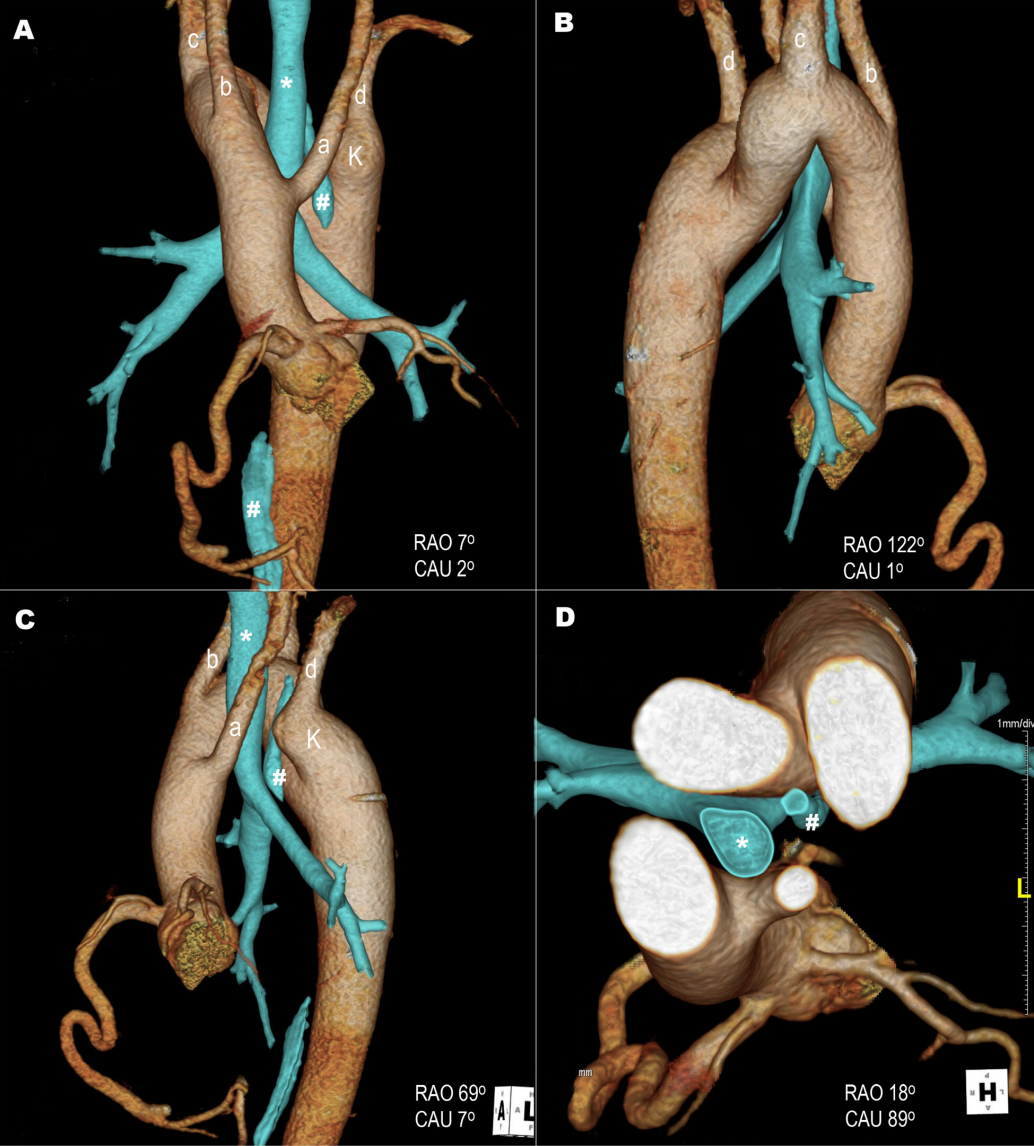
Three-dimensional CT angiography images. Relationships of right-sided aortic arch and its branches with trachea (*) and esophagus (#) in different directions (A-D). Left common carotid artery (a), right common carotid artery (b) and right subclavian artery (c), originated separately in said order. Left subclavian artery (d) separately arises from a Kommerell’s diverticulum (K). RAO indicates right anterior oblique; CAU, caudal.
